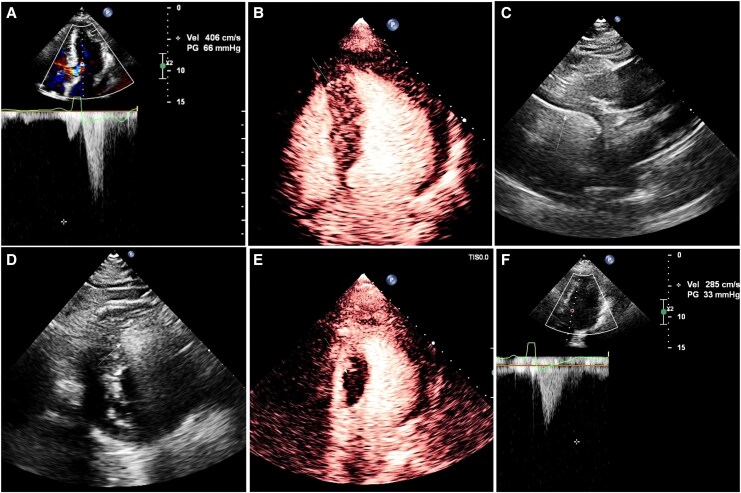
Imaging examinations before, during, and after surgery. (*A*) Pre-operative transthoracic echocardiography demonstrating left ventricular outflow tract obstruction. (*B*) Myocardial contrast-enhanced echocardiography showing severe septal hypertrophy (thickness 22 mm). (*C*) The position of the radiofrequency ablation needle within the basal anterior septum was confirmed utilizing multiple echocardiographic views. (*D*) Arrow indicates the hyperechoic gas-bubble zone following radiofrequency ablation. (*E*) Post-ablation myocardial contrast-enhanced echocardiography revealing a contrast filling defect within the ablation zone. (*F*) Post-operative transthoracic echocardiography demonstrating a left ventricular outflow tract gradient of 33 mmHg.
